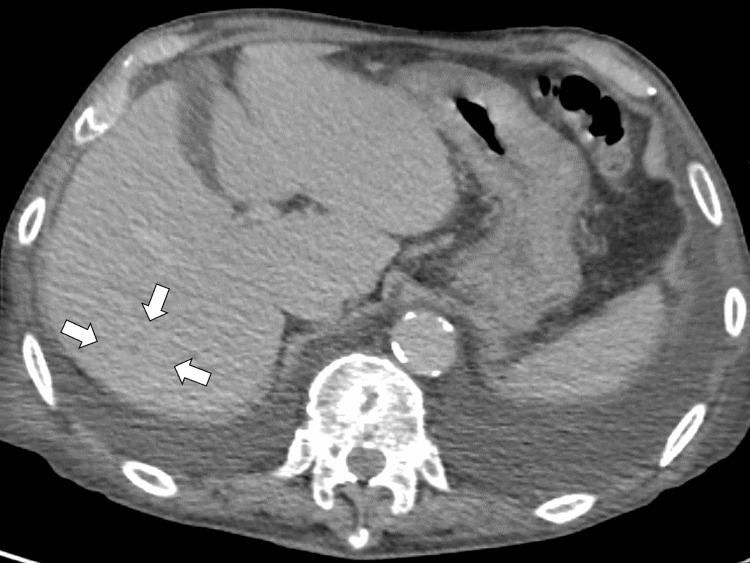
Repeat contrast-enhanced computed tomography showing further reduction of the hepatic abscess and no new infectious foci (white arrows).
